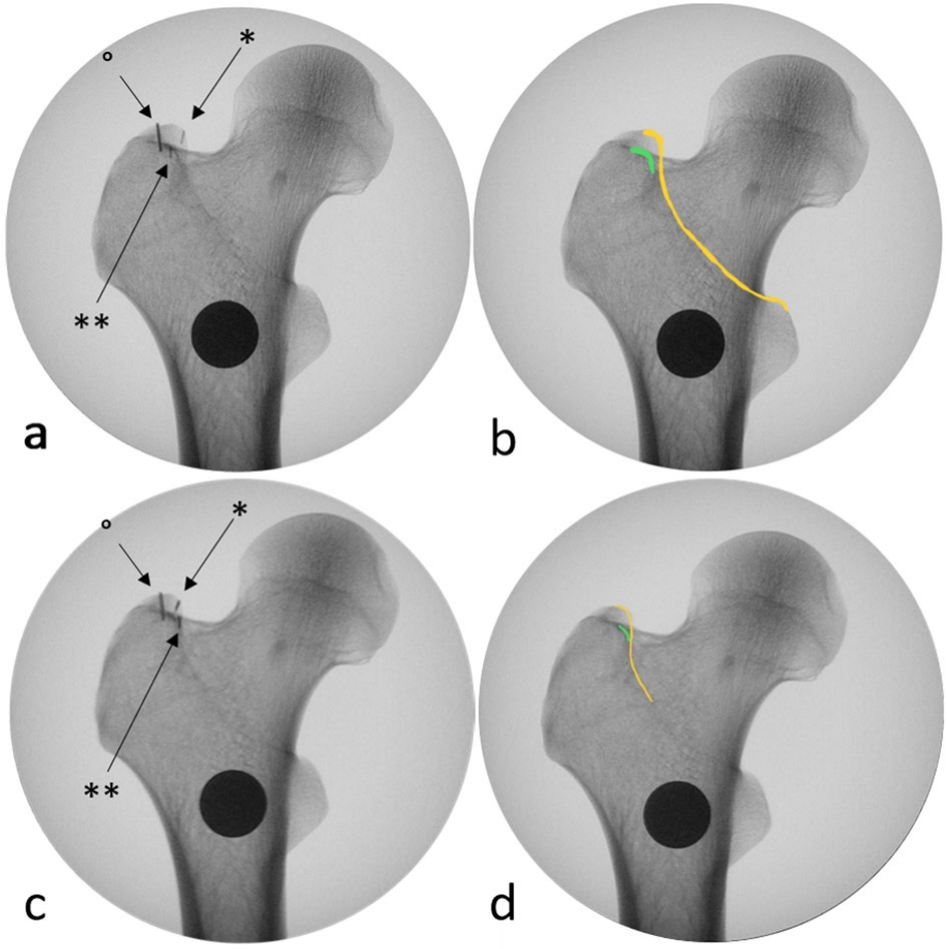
Defining the radiological landmarks. (a,b) and (c,d) are each pairs of an identical radiological view of the proximal femur. (c,d) both show the COV while (a,b) show an ap view, which deviates from the COV by 10° external rotation. (a) xray image with markers indicating the postero-superior border of the GT (*) and the anterio-superior border of the GT () as well as the tip of the GT (°). (b) the postero-superior border of the GT is the extension of the intertrochanteric crest (yellow). A density line of the piriform fossa is easily recognized (green). (c,d) the antero- and postero-superior borders of the GT overlap in the COV, this coincides with an overlapping of the easily recognizable intertrochanteric crest and density line of the piriform fossa.
